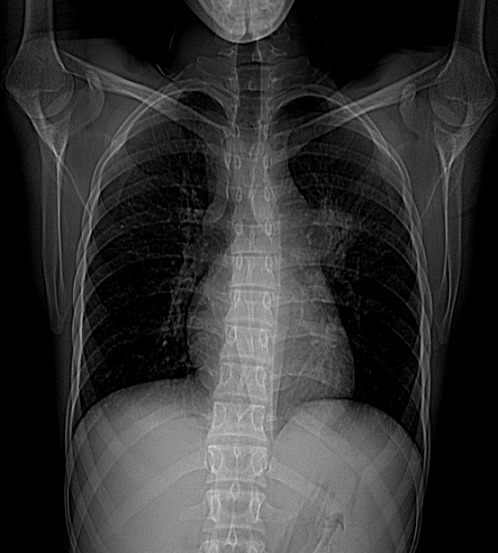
Radiographie thoracique de face montrant une opacité hilaire gauche grossièrement arrondie dont la limite interne est noyée dans l’opacité médiastinale, la limite externe relativement convexe, élargissement médiastinal
